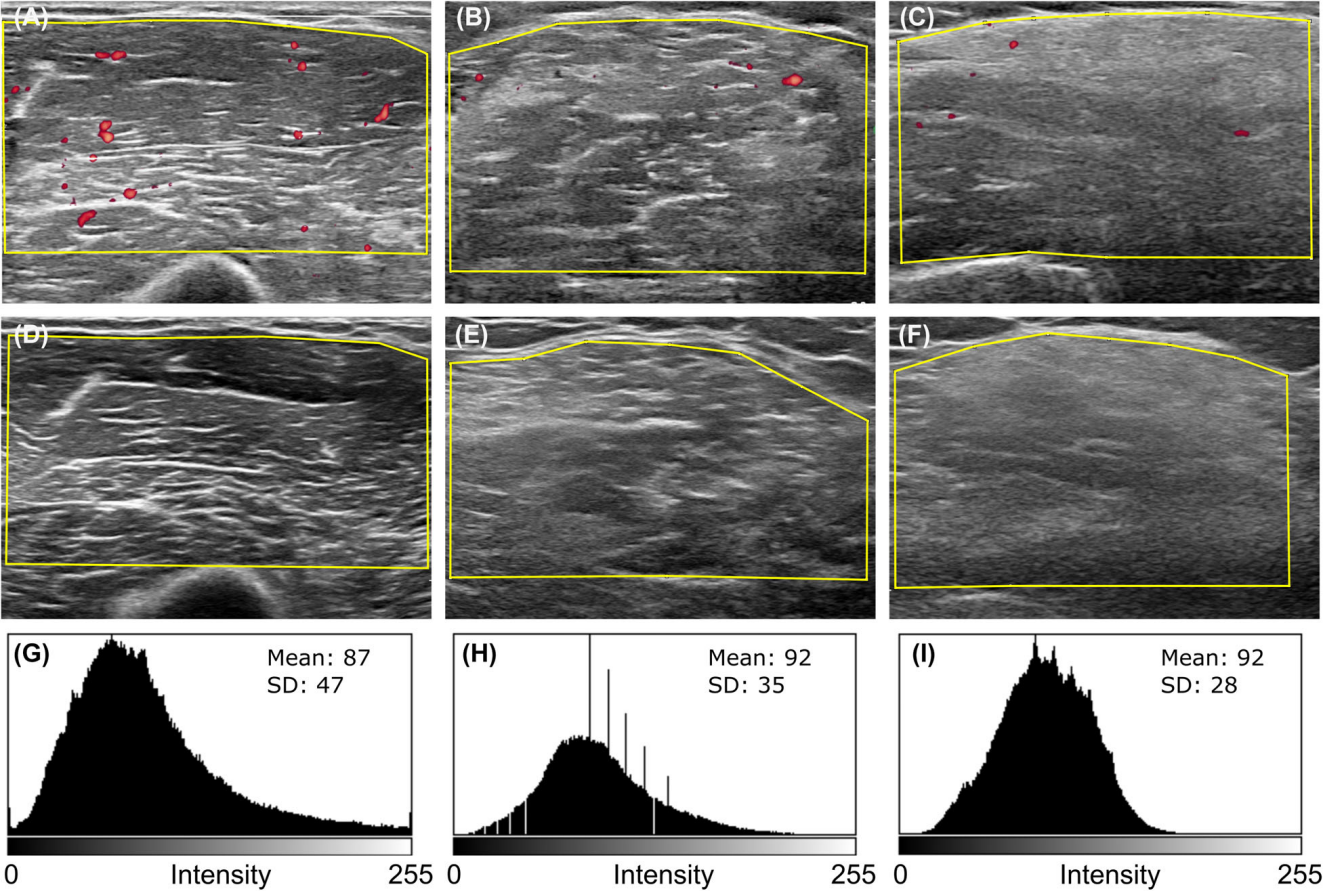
Power Doppler and ultrasound imaging of the elbow flexor muscles. A healthy control muscle (A) shows prominent blood flow, while this is reduced in a BMD patient (B), and in a patient with a dysferlinopathy (C). The echotexture of a normal muscle is heterogeneously speckled (D), attenuated in muscular dystrophy patients (E and F). Histogram analysis of the corresponding above‐placed photos shows the echotexture distribution pattern, the mean echointensity value, and the standard deviation. In contrast with the healthy control (G), both BMD (H) and dysferlinopathy (I) patients show increased mean and reduced standard deviation of echointensity. The X axis of the histograms represents echointensity values between 0 and 255, and the Y axis represents the frequency of observations. BMD, Becker muscular dystrophy; SD, standard deviation.
